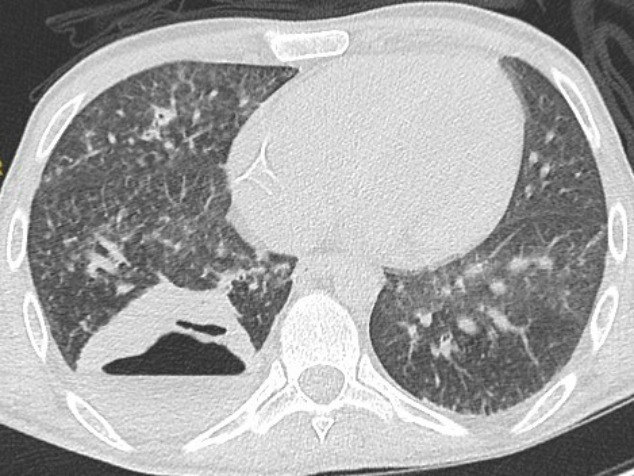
CT scan of chest 7 days post-admission. A 73×69 mm thick walled abscess cavity with a fluid level is seen in the right lung.
